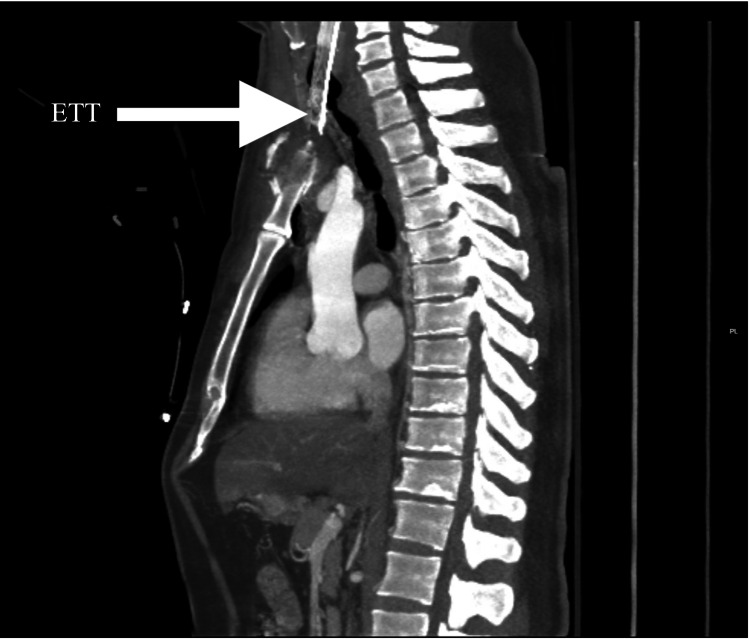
Sagittal CT chest showing anterior perforation of endotracheal tube (ETT) through the tracheal injury
